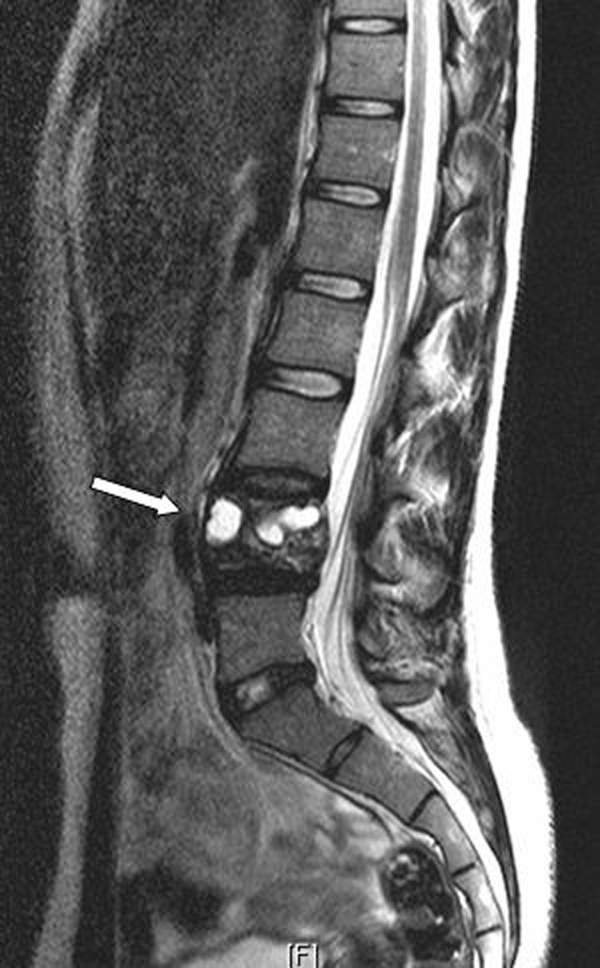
Sagittal T2-weighted MRI demonstrating cystic components of vertebra L4.
